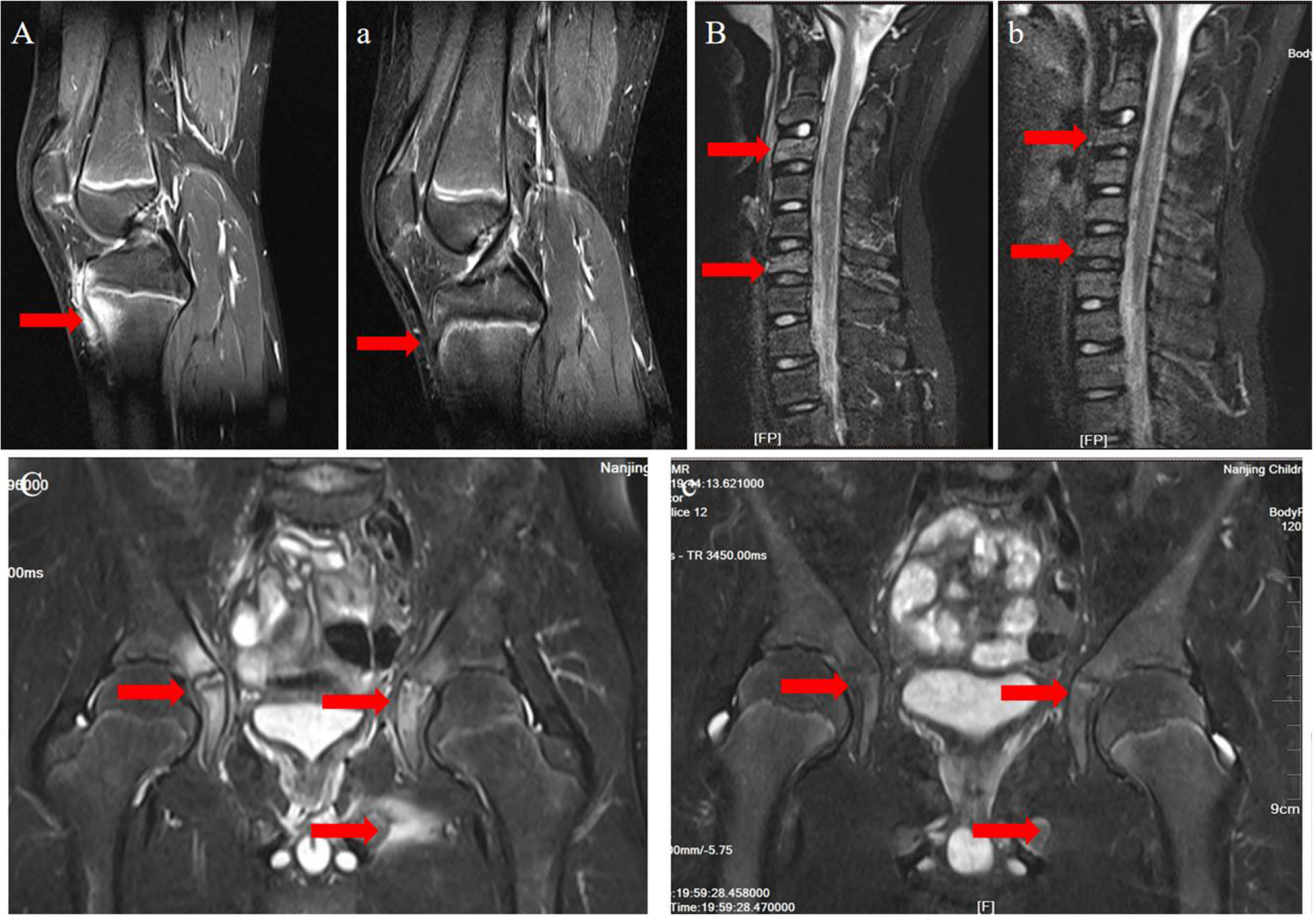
MRI findings in case 18 with chronic nonbacterial osteomyelitis MRI STIR or T2 show hyperintensity before treatment in tibia tubercle (A), cervicle vertebra (B) and hip joint (C), and remission after treatment (a, b, c)
